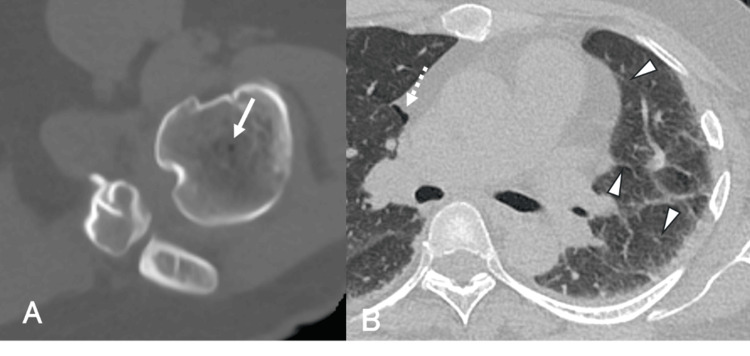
Case 3 Pre-hyperbaric oxygen therapy (HBOT) plain computed tomography (CT) images. A: Bone-window settings (window width of 3000 HU and a window level of 400 HU). B: Lung-window settings. An intramedullary air bubble is observed in the left proximal humerus (solid arrow in A), and a mediastinal bubble is observed on the right side (dashed arrow in B). Thickening of the interlobular septa in the left lung field suggests pulmonary congestion (arrowheads in B).
